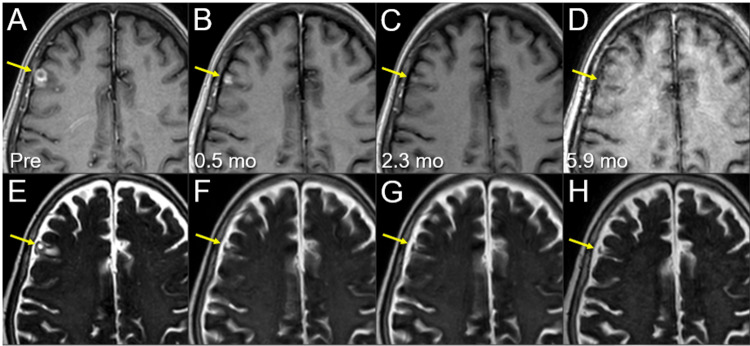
Magnetic resonance images before and after the third 10-fr SRS combined with simultaneous whole-brain irradiation for local failure following prior SRS of the right frontal lesion. The images show (A-D) axial CE-T1-WIs and (E-H) axial T2-WIs (A, E) five days before (Pre) the third SRS (17.3 months after the initiation of CRT); (B, F) at 0.5 mo after the third SRS; (C, G) at 2.3 months; and (D, H) at 5.9 months after SRS (23.9 months after CRT). (A-H) These images are shown at the same magnification and coordinates under co-registration and fusions. (A-H) The solid enhancing lesion (arrows in A and E) shrank remarkably from 0.5 to 2.3 mos (arrows in B, C, F, and G) and almost disappeared at 5.9 mos (arrows in D and H). The pre-existing perilesional edema (arrow in E) disappeared without the development of any adverse radiation effects (arrow in H) at 5.9 mos. CE, contrast-enhanced; WIs, weighted images; SRS, stereotactic radiosurgery; CRT, chemoradiotherapy; mo, month; fr, fraction
